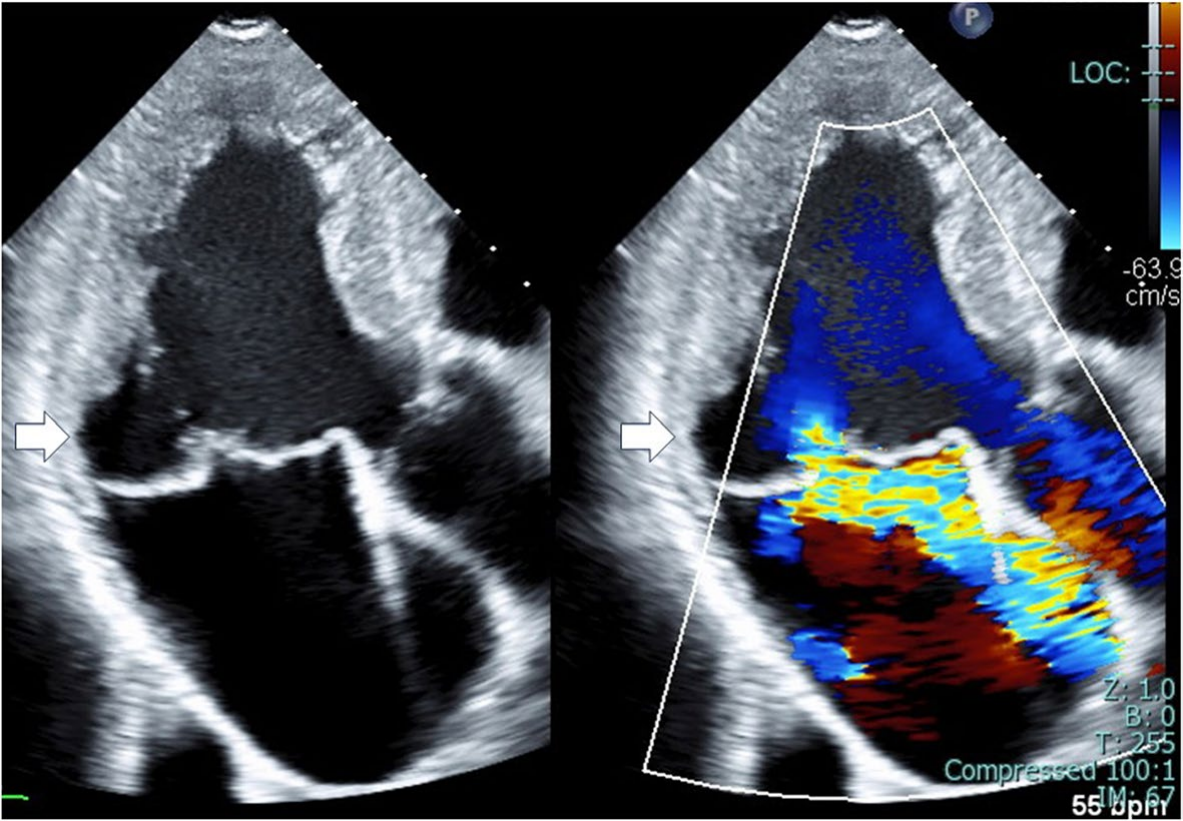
Transthoracic echocardiograms show an enlarged mitral annulus, mitral valve billowing with posterior leaflet prolapse and mitral annular disjunction (white arrows), and severe mitral regurgitation
